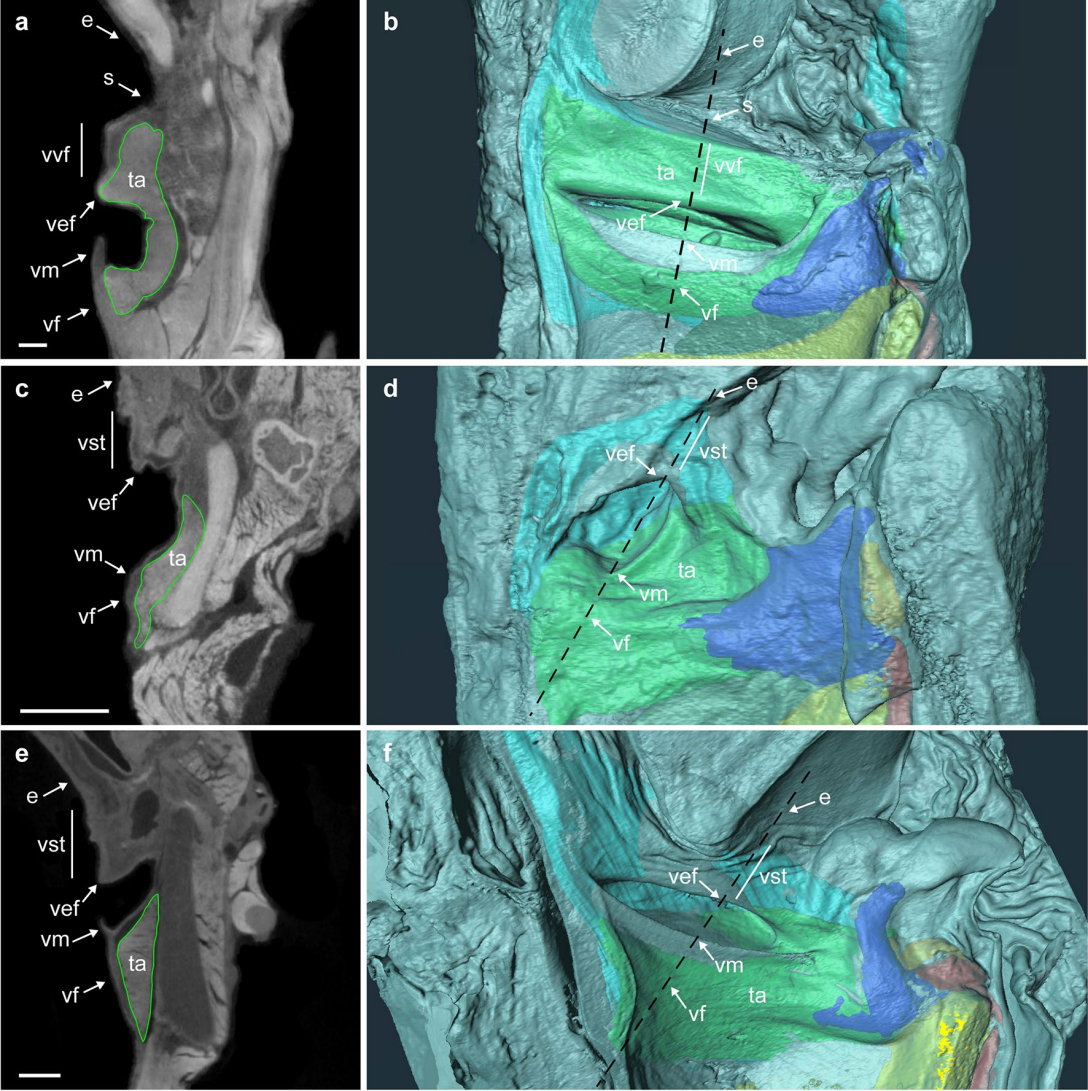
Gross-anatomy of the larynx. (a, b) CT frontal scans and 3D images of *Lemur catta* (lemuriform); (c, d) *Nycticebus coucang* (lorisiform); and (e, f) *Macaca fuscata* (anthropoid). Scale 1 mm. The cutting planes of (a), (c), and (e) are the dashed lines on (b), (d), and (f), respectively. e, epiglottis; s, sulcus between the vestibule and the epiglottis; ta (green), thyro-arytenoid muscle; vef, vestibular fold; vf, vocal fold; vm, vocal membrane; vst, vestibule; and vvf, vestibular vocal fold. dark blue, arytenoid cartilage; yellow, cricoid cartilage; red, posterior cricoarytenoid muscle; and light blue, thyroid cartilage.
